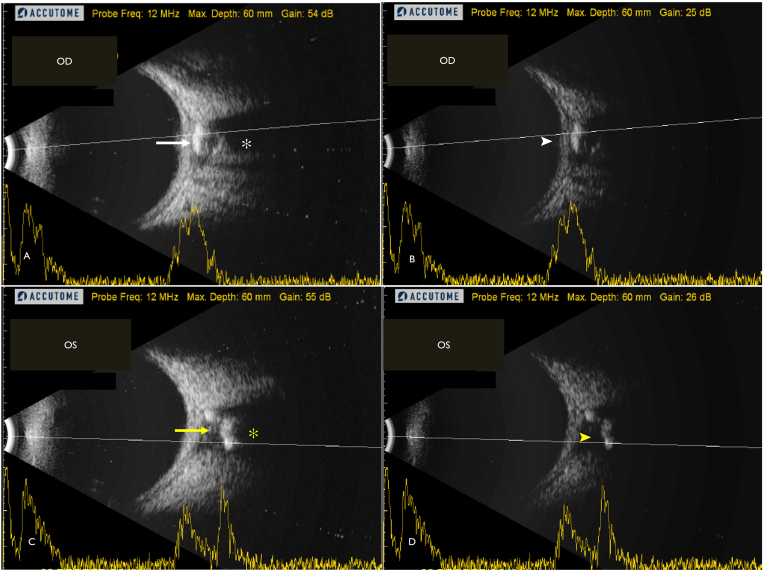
(A, C) Ultrasound-B scan of the right eye shows a hyperechoic lesion present posterior to optic nerve head and in the peripapillary area (solid white arrow) with posterior acoustic shadowing (white asterix) in the right eye (OD) and the left eye (OS) (solid yellow arrow and yellow asterix) respectively. (B, D) Ultrasound-B scan of the right eye (OD) and the left eye (OS) shows persistence of hyper echogenicity (white and yellow arrow-head) at adjusted low gain of the ultrasound B scan. (For interpretation of the references to color in this figure legend, the reader is referred to the Web version of this article.)
